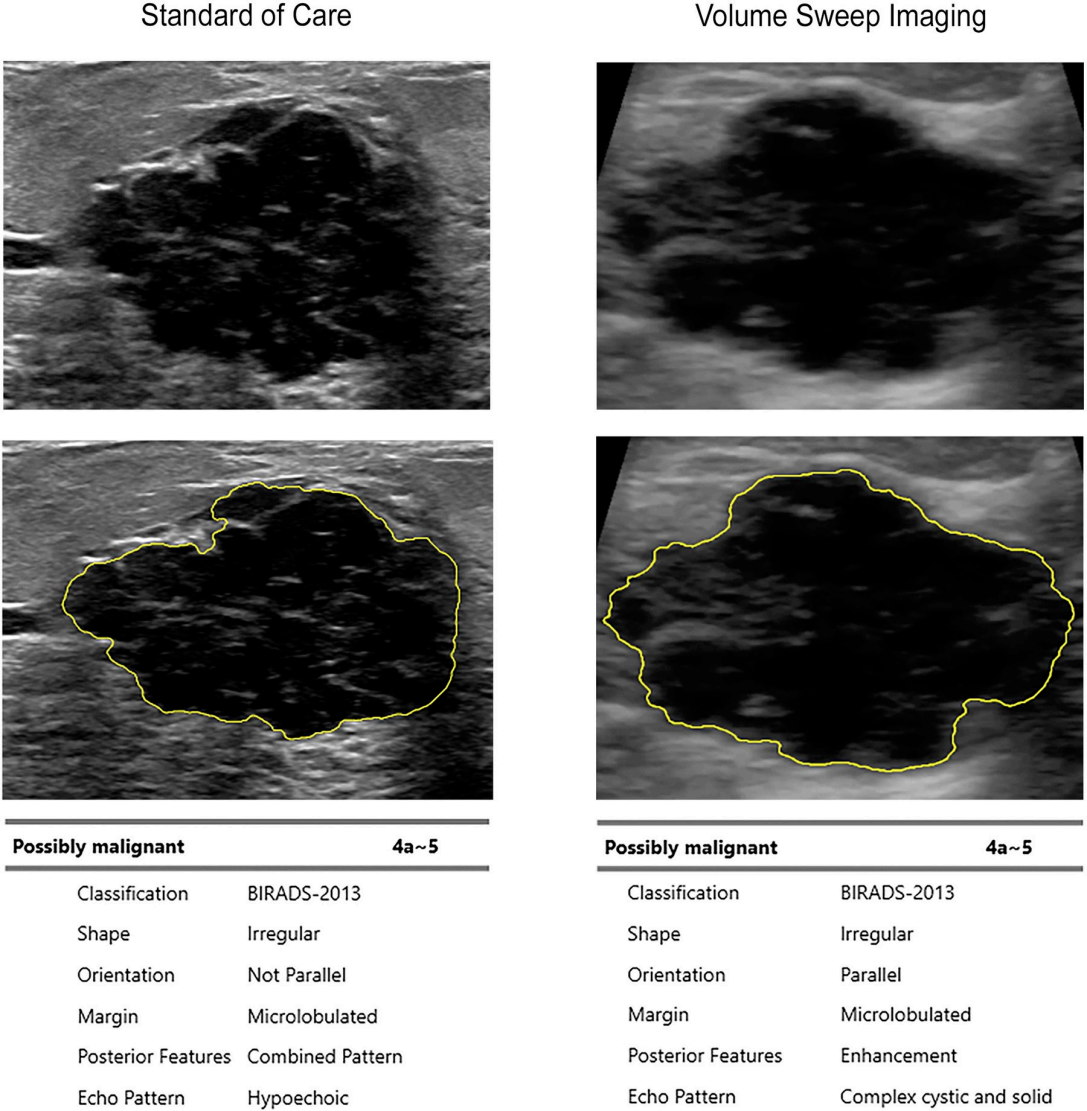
S-Detect Interpretation of Pathology Proven Invasive Ductal Carcinoma on Standard of Care and VSI. B-mode ultrasound images of invasive ductal carcinoma are shown with their respective mass segmentations and S-Detect produced reports. The standard of care image was obtained by a certified expert breast sonographer using a Philips Epiq 7G ultrasound machine. The VSI image was obtained by a medical student without prior medical experience after less than 2 hours of training on a portable Butterfly iQ ultrasound probe. S-Detect results between standard of care and VSI are similar with ultimate agreement on the “possibly malignant” interpretation. The S-Detect results were also similar to expert interpretation of standard of care and VSI with ultimate agreement on the “possibly malignant” characterization. The original BI-RADS assessment on both VSI and standard of care imaging expert interpretation was 4 (biopsy warranted). There was complete agreement across expert interpretation and S-Detect on microlobulated margins.
